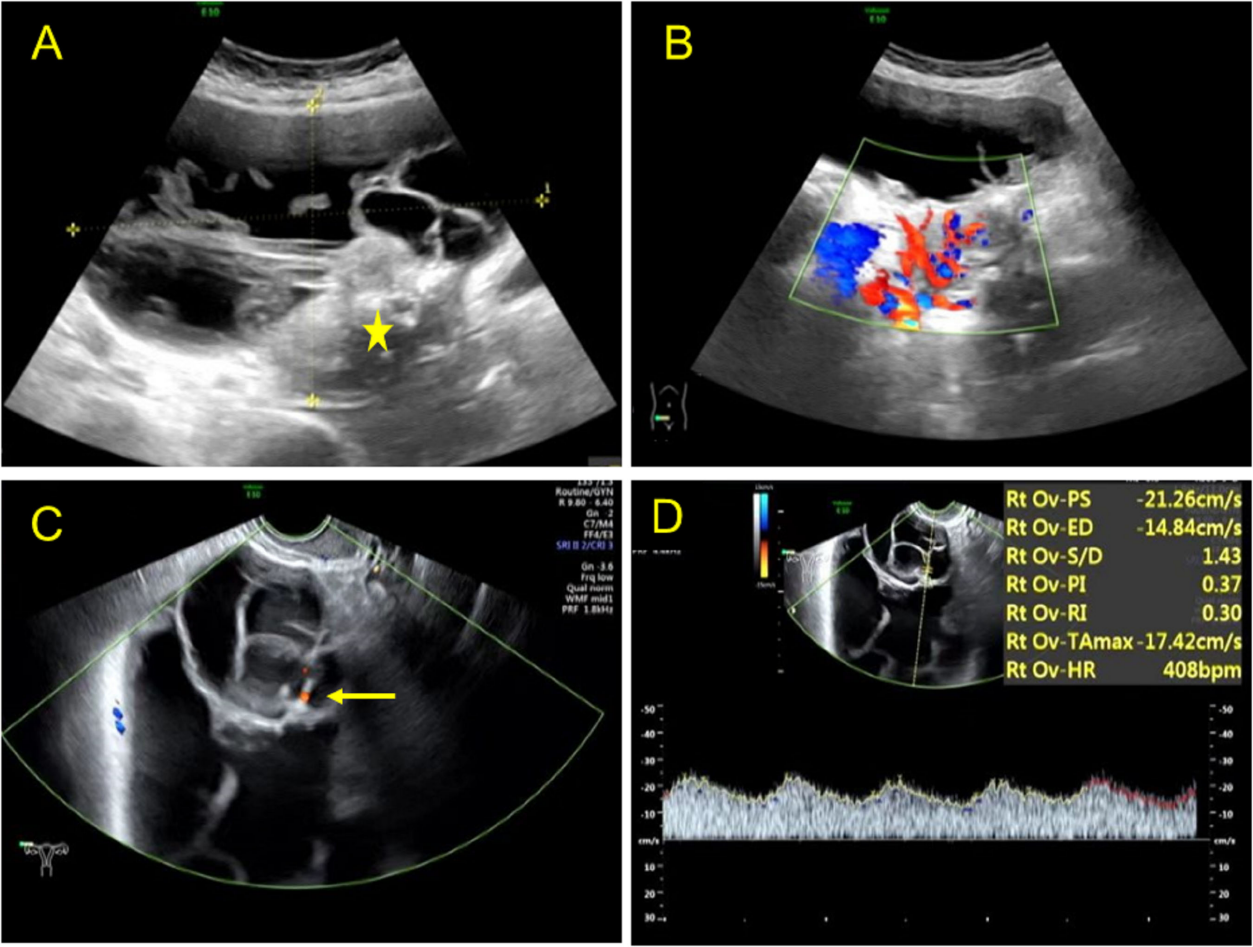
Ultrasound images of a benign Brenner tumor patient of the mixed cystic and solid type. (A) Transabdominal sonography showing a giant mixed cystic and solid (asterisk) lesion in the right lower abdomen with a diameter of 15.1 × 9.8 cm. (B) Color Doppler imaging revealed an abundant blood flow in the solid part of the lesion. (C) Transvaginal sonography showing a few flow signals in the separates with color Doppler (arrow). (D) Pulsed wave Doppler demonstrating a low resistant index of blood flow in the lesion.
